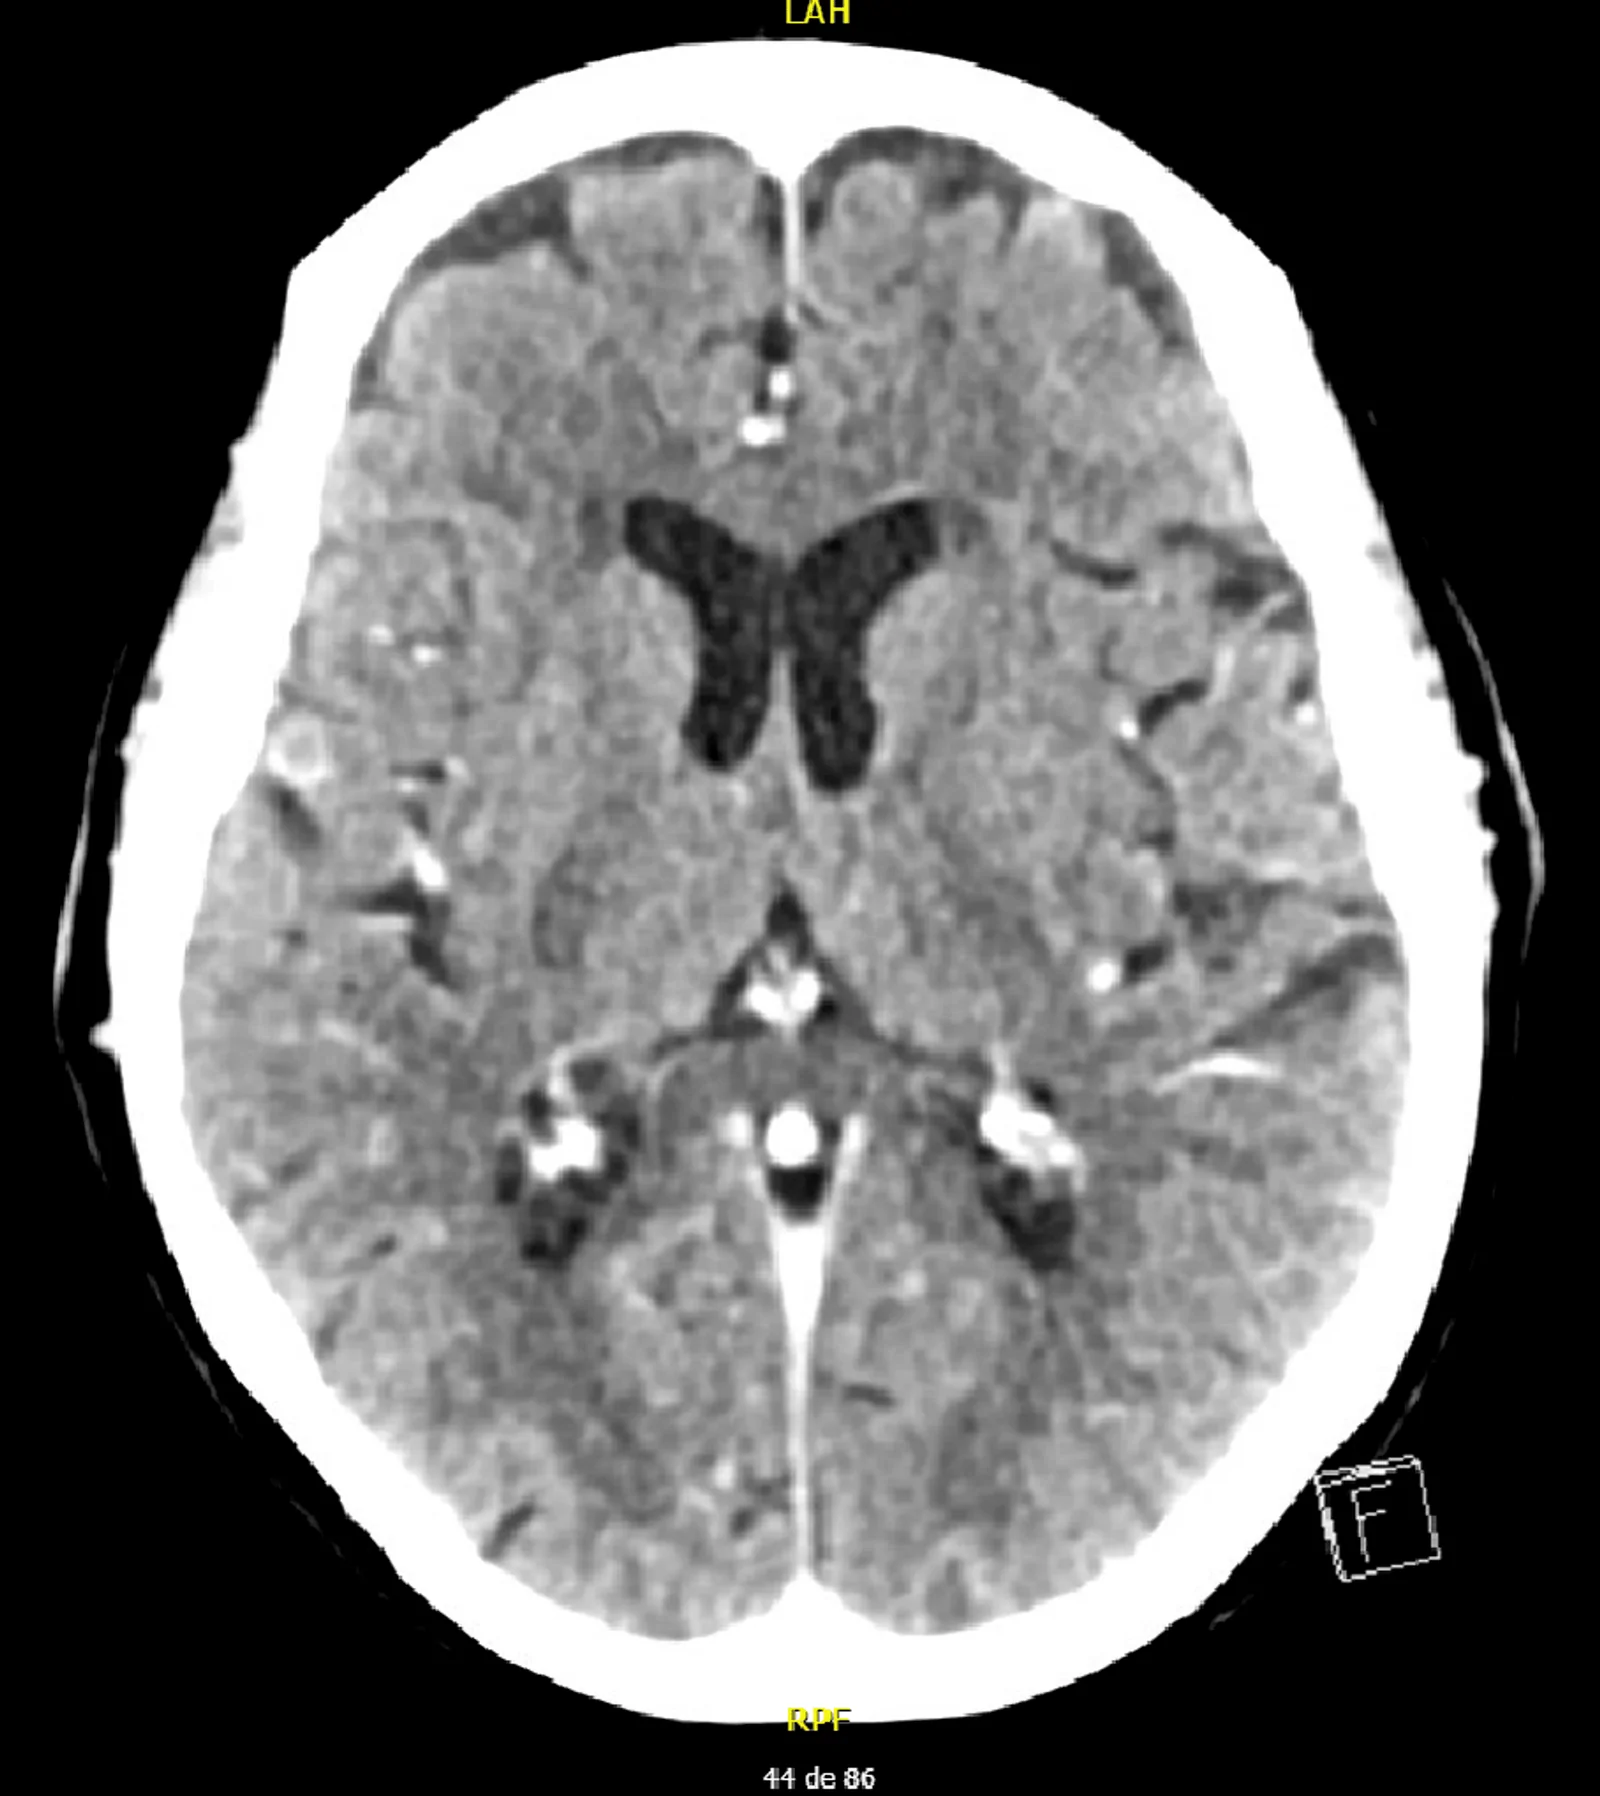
Transversal section of the computed tomography angiography
